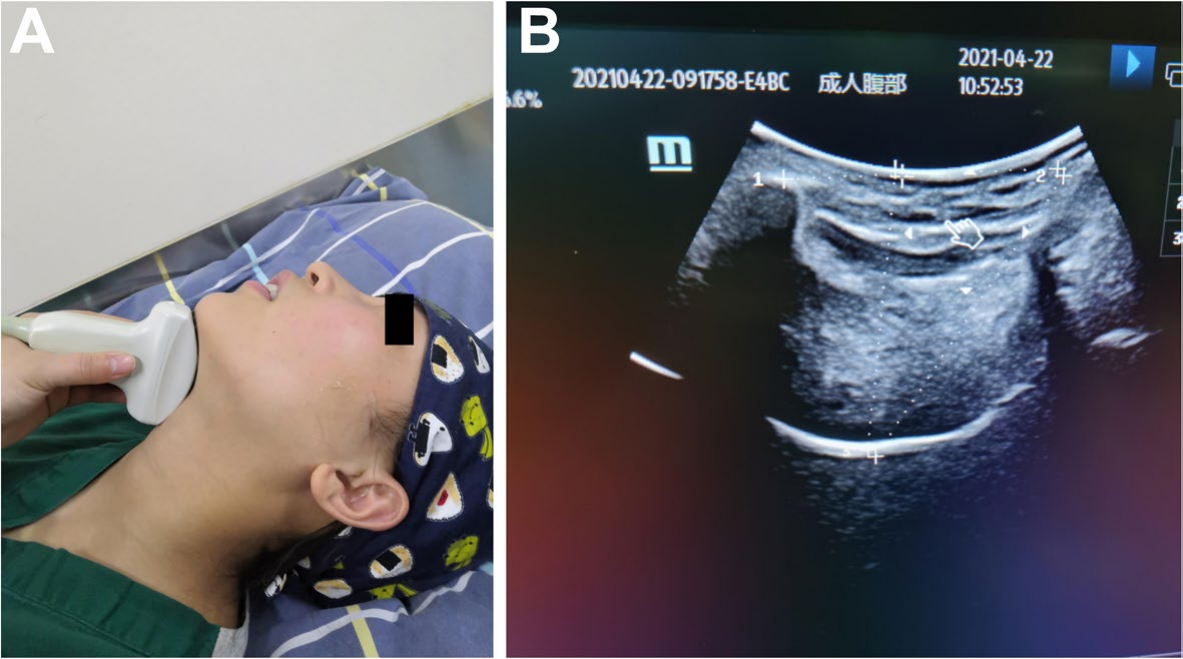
The method of measuring the hyomental distance and tongue thickness under ultrasound. The body surface position of the ultrasound probe (A) and imaging (B). D12 represents the hyomental distance, which is 52.31 mm. D34 represents the tongue thickness, which is 46.83 mm
